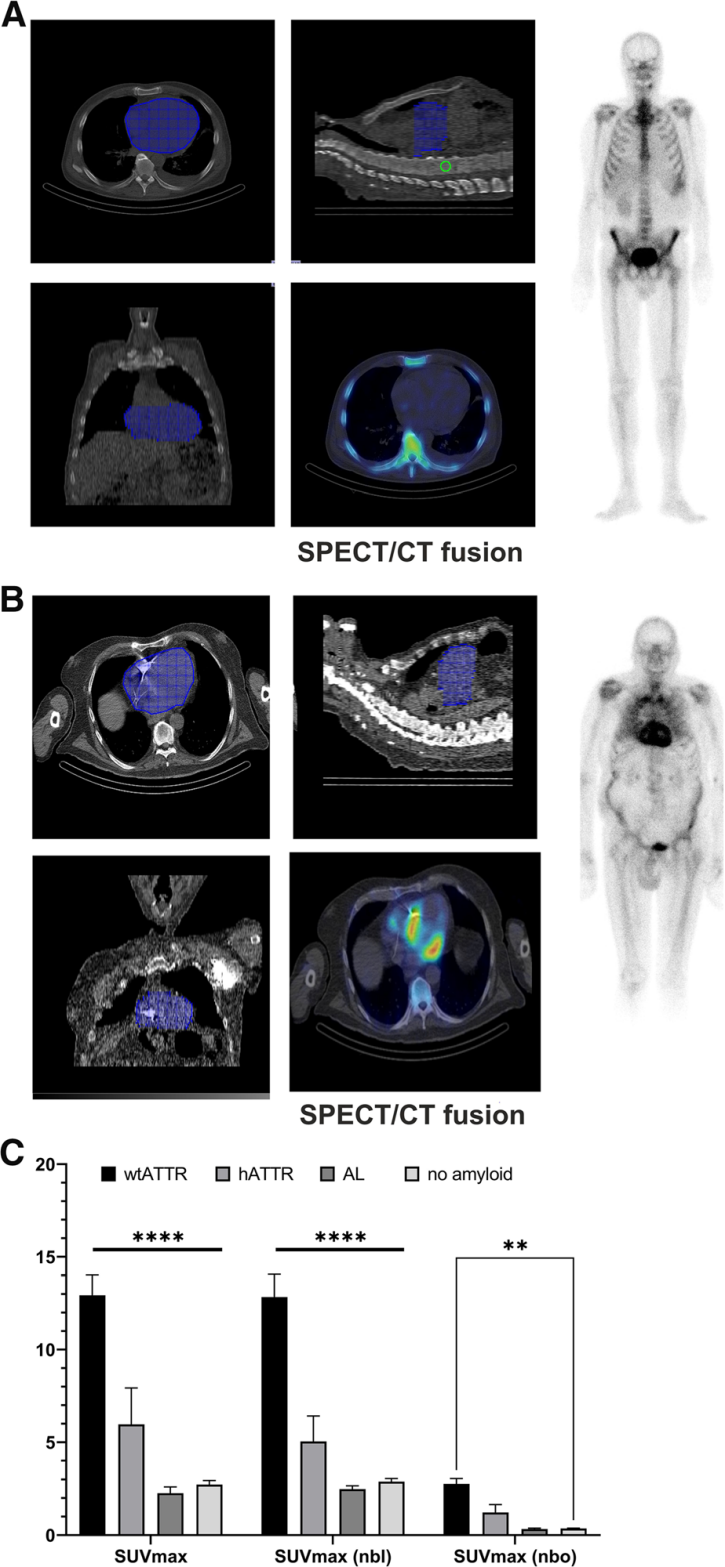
Representative example cases. VOI delineation in blue. (A) 66-year-old male with AL amyloidosis does not show any cardiac uptake in planar scintigraphy (Perugini 0) and in SPECT/CT images with a SUVmax of 1.6. (B) 84-year-old male with wtATTR amyloidosis and Perugini score 3 in the planar images with decreasing uptake in bone. SPECT/CT shows high tracer uptake in the left ventricular myocardium and a SUVmax of 26.7. (C) Difference in cardiac uptake of amyloidosis subtypes for the three SUV parameters. Patients with wildtype ATTR amyloidosis exhibited significantly higher uptake values compared (*p* < 0.001)
